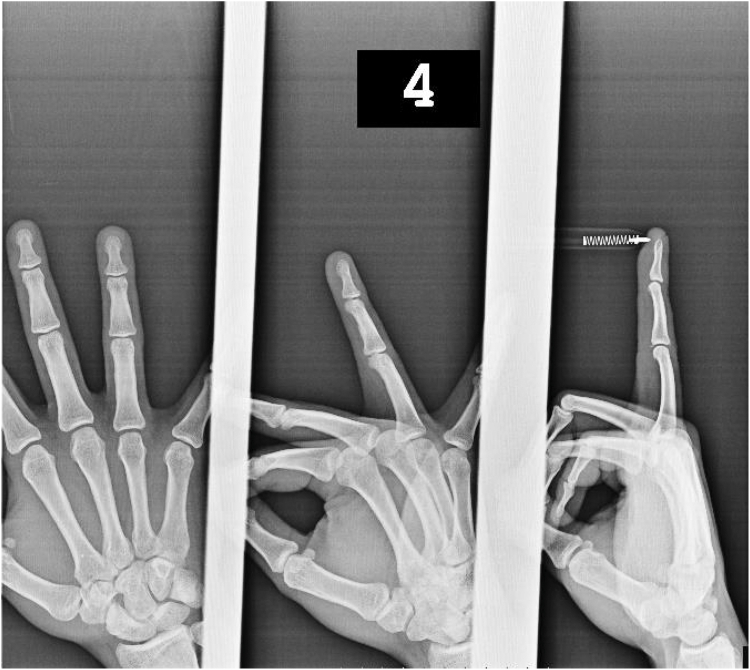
Control lateral radiograph of the right fourth finger obtained prior to application of the 30° flexion circular plaster cast.
